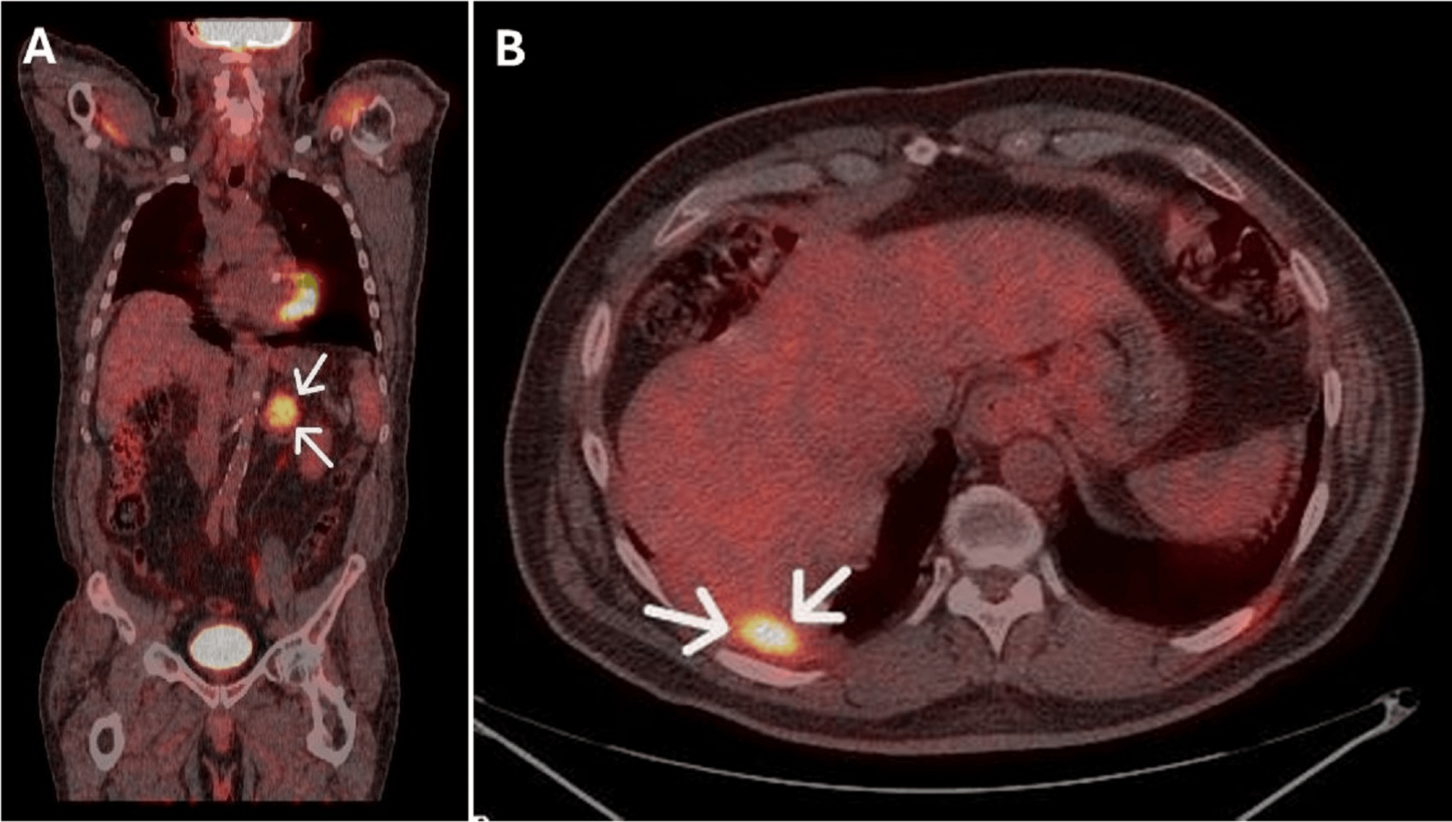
The fluorodeoxyglucose positron emission tomography (FDG-PET) scan of body from neck to thigh. A: Demonstrating high tracer uptake in the left adrenal gland lesion on coronal view (white arrows). B: Demonstrating high tracer uptake in the right subpleural mass and pleural thickening on transverse view (white arrows).
